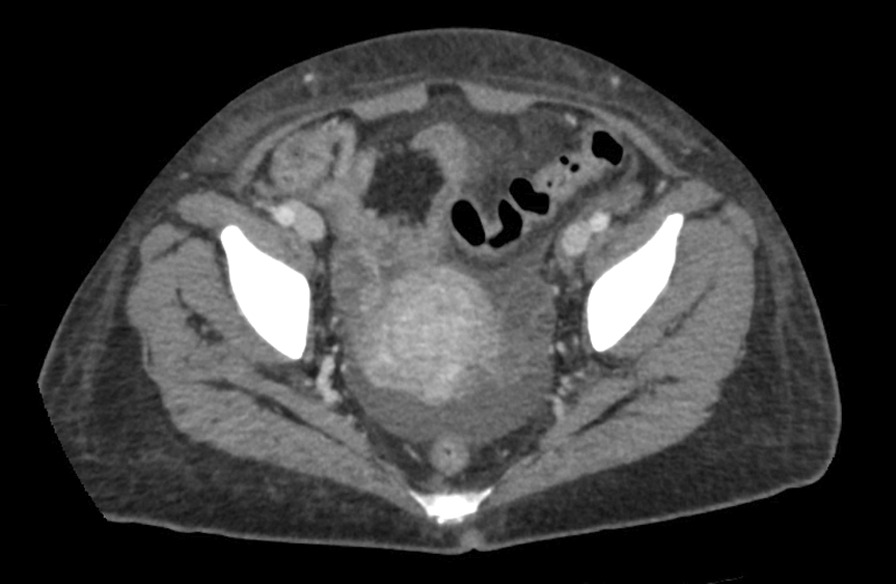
Computed tomography (CT) of the abdomen and pelvis with contrast was obtained revealing an approximately 3.5 × 3.0 cm heterogeneously enhancing mass protruding into the endometrial canal favored to represent an intracavitary submucosal leiomyoma
